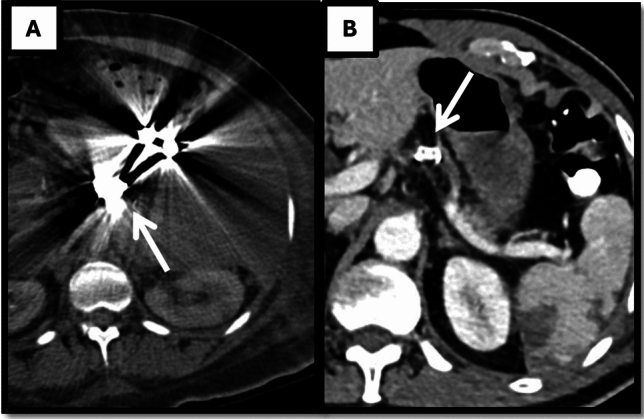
A Axial unenhanced CT abdomen of a 49-year-old male following coil embolization of an idiopathic splenic artery aneurysm demonstrating extensive streak metallic artifacts from the coils (arrows) that obscure the diagnostic quality of the image. B Axial CECT abdomen of a 66-year-old male following splenic artery embolization with a LOBO device, demonstrating the device in situ with no significant metallic artifact and clear delineation of the surrounding parenchyma (arrows)
